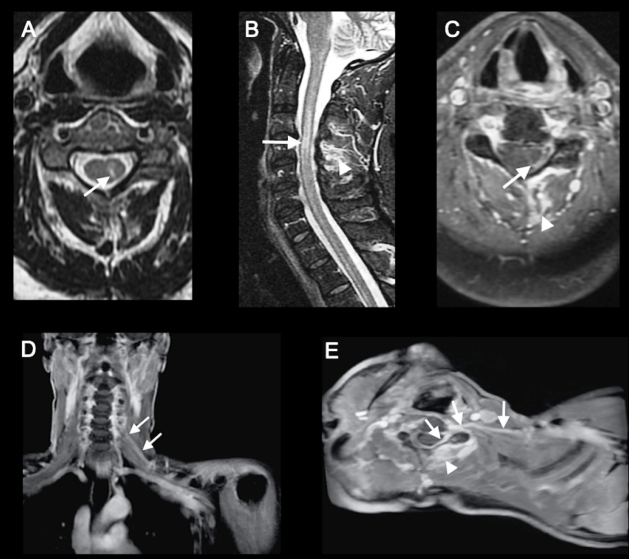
The reactivation of varicella zoster virus is complicated by myelitis and radiculitis. Cervical spine MRI showing hyperintense signal in the left gray matter columns of the spinal cord at C3 to C5 based on axial T2-weighted imaging (arrow in A) and sagittal STIR (arrow in B), and with contrast enhancement (arrow in C). Contrast enhancement was also detected in the left C5 spinal nerve root and the nerve (arrows in D and E), suggesting myelorradiculitis. The edema and contrast-enhancement in the corresponding homolateral paravertebral muscles (arrowheads) are of additional note.
